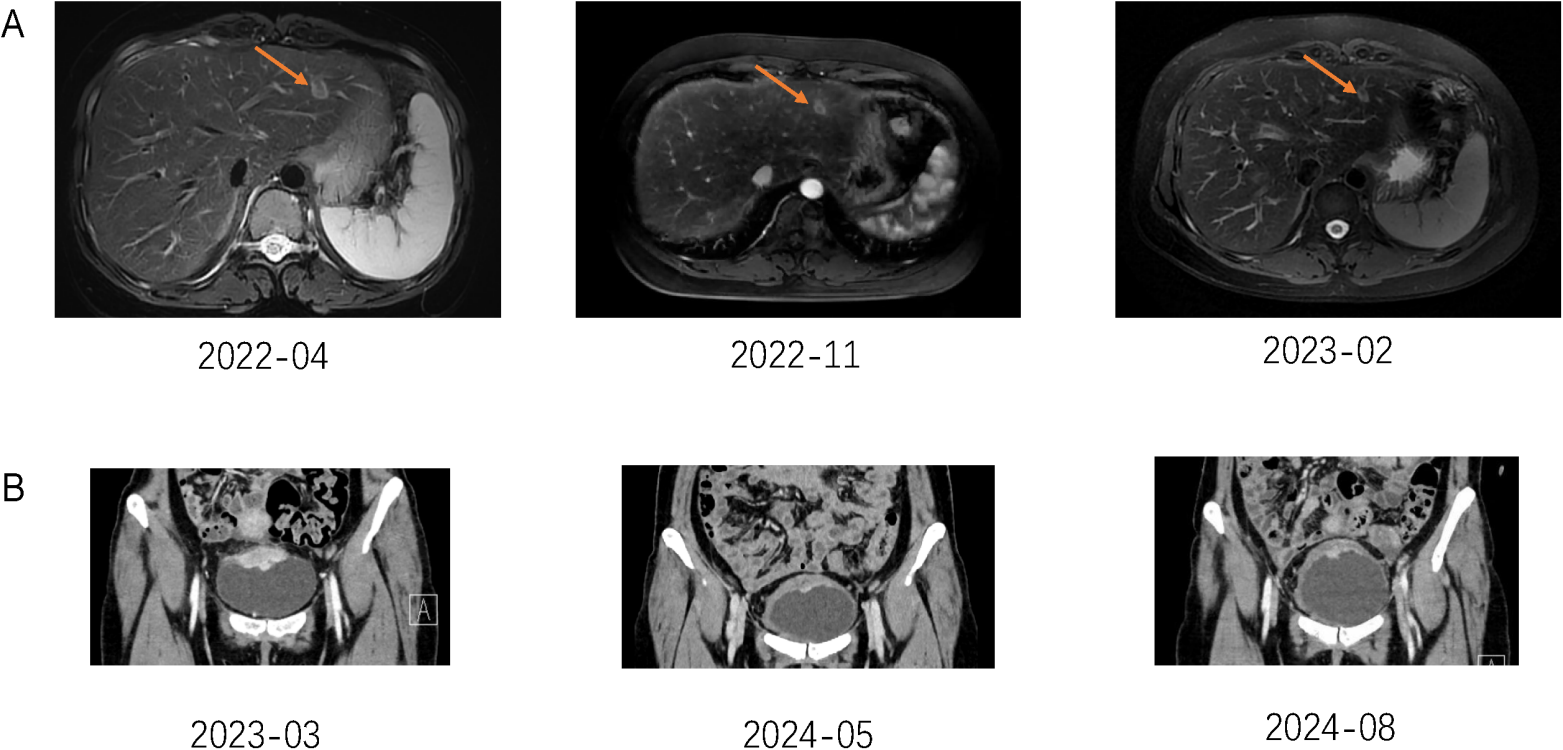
(A) The Orogen arrow in the figure shows liver metastases. (B) Pelvic MRI examination results suggest that bladder metastases have shrunk.
